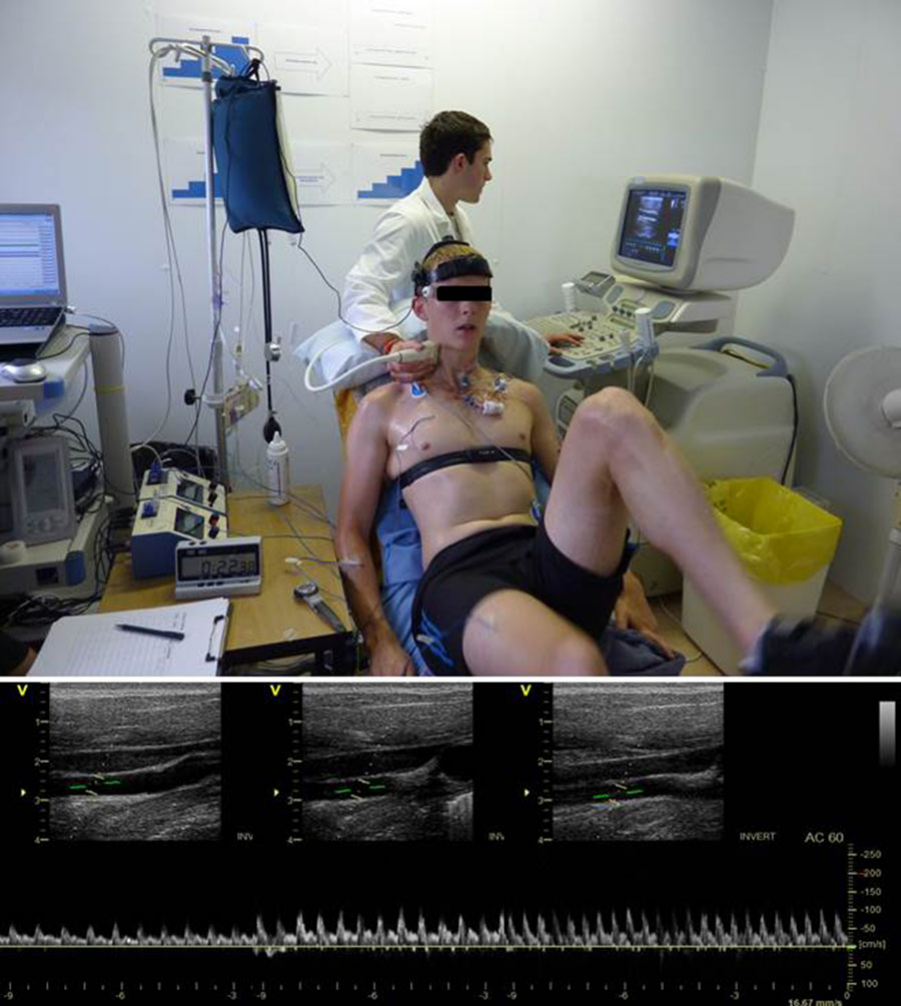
Experimental arrangement and ultrasound recording Photo depicting one of the participants in the study performing an incremental cycling test on a semi-recumbent cycle ergometer (Lode Angio, Groningen, the Netherlands) with a backrest inclination of 45 deg, while measurements of ICA and CCA blood flow were obtained at each stage. Representative images of real time ICA blood velocity recordings at rest, submaximal and peak exercise are shown.
